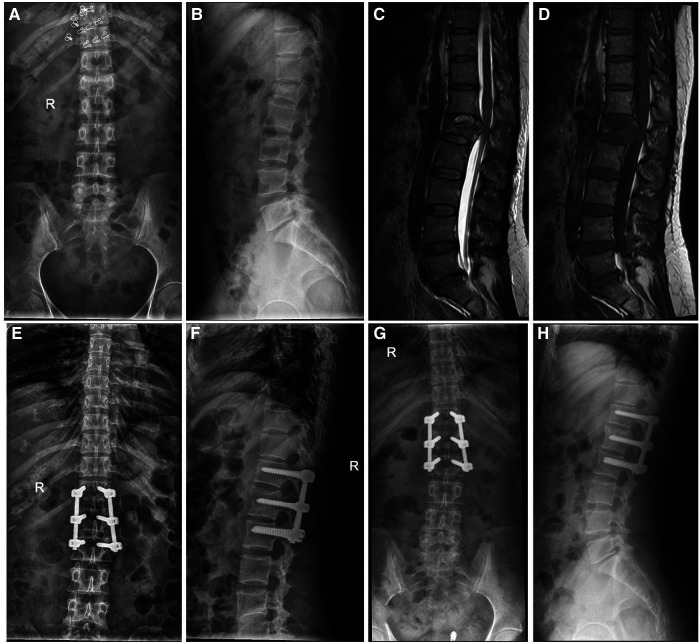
A 40-year-old female patient with L1 fracture underwent short-segment fixation with bilateral pedicle screw fixation in the fractured vertebra. (A–D) The preoperative x-ray and MRI images showed burst fracture of the L1 vertebra. The compression degree of the injured vertebra was 40%. (E,F) x-ray examination, conducted immediately after surgery, showed that the height of the L1 vertebra was recovered and the kyphosis was corrected. (G,H) Postoperatively (1.5 years), x-ray examination showed that the height of the L1 vertebral body was well maintained.
